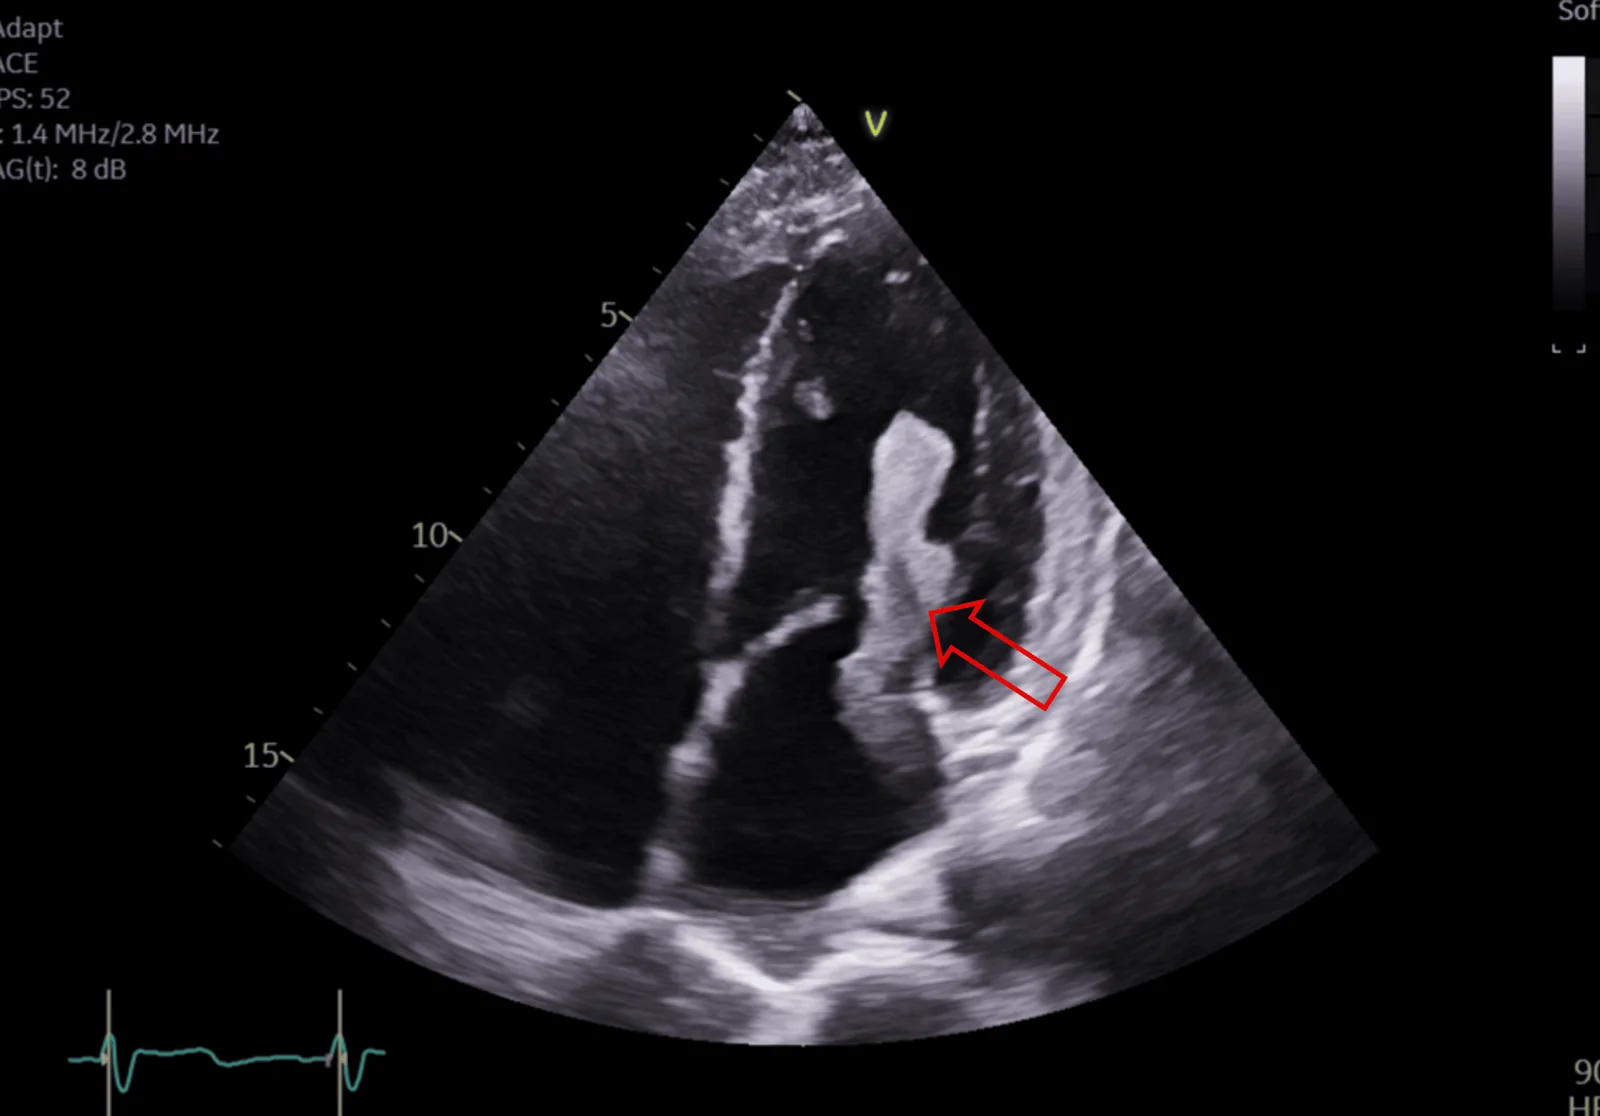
Transthoracic echocardiography (apical four-chamber view), demonstrating a giant multilobulated left atrial mass prolapsing into the left ventricle.
